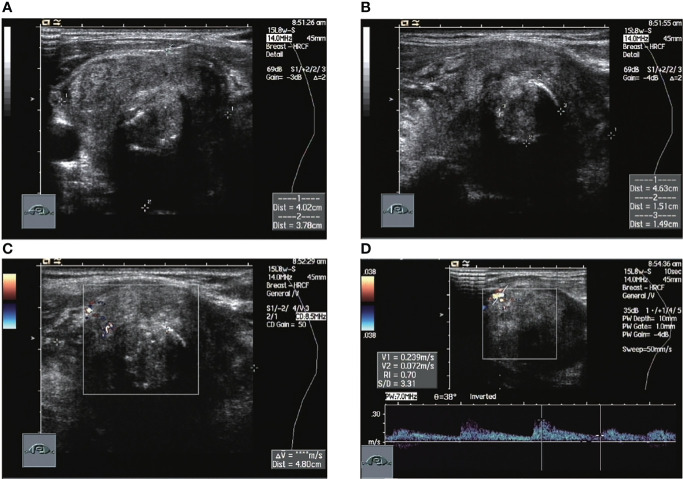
Ultrasound images of patient #5 in which normal thyroid tissue is no longer visible. The nodule occupies the entire image, with annular calcifications visible within it and a relatively rich local blood flow signal of RI=0.7. This patient had a recurrence 2 months after surgery, was treated with radiation after a second operation and was finally lost to follow-up. (A) Sagittal scan (B) Transverse scan (C) CDFI (D) Pulsed doppler.
